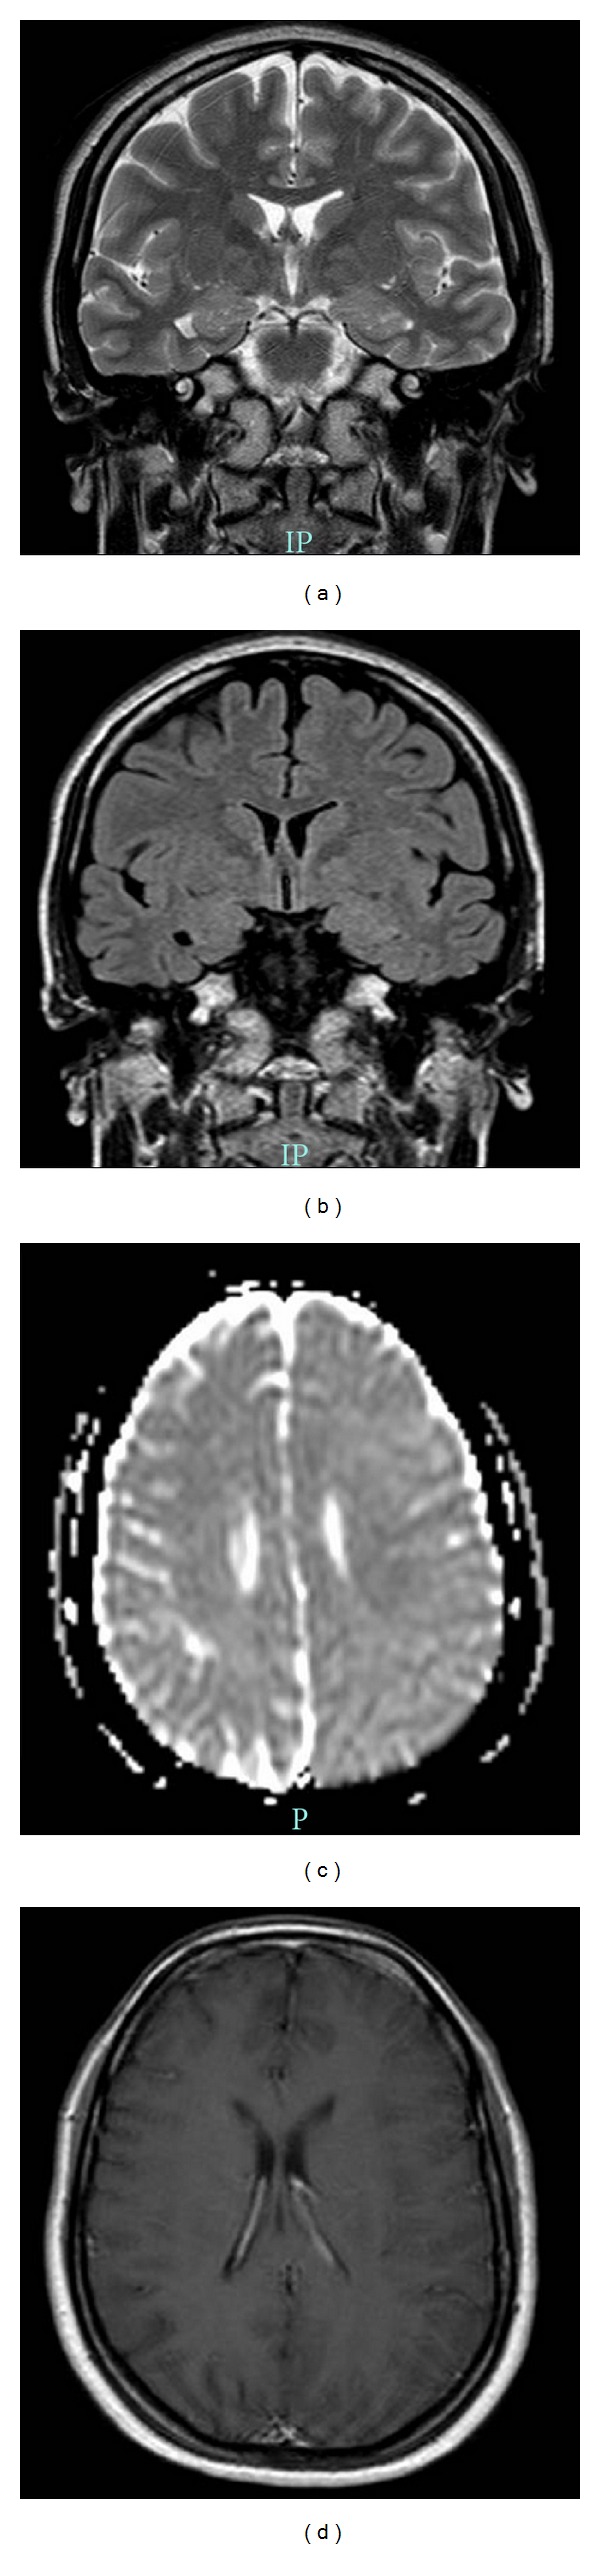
MRI of the patient at the time of presentation. (a) Transverse fast spin-echo T2-weighted MR images through the level of the sylvian fissures show diffuse cortical swelling and mild cortical hyperintensity of the left cerebral hemisphere. These findings are most likely caused by cortical edema. (b) Coronal diffusion-weighted MR image through the level of the basal ganglia shows diffuse high signal of the cortex of the left cerebral hemisphere. (c) Diffusion-weighted images show no evidence of water restriction in the left hemisphere. (d) Postcontrast study shows that the area does not enhance on T1-weighted images after contrast medium.
